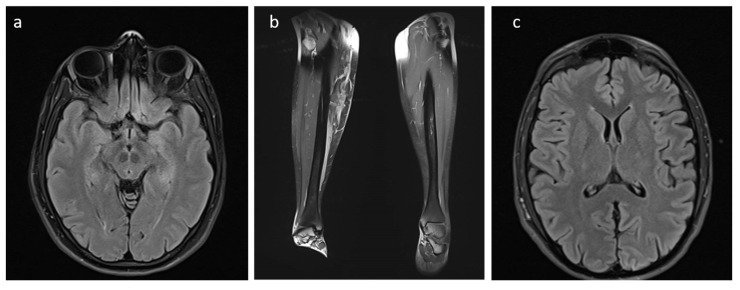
MRI of patients 1 (a,b) and 2 (c). Patient 1: Hyperintensity lesions with nonspecific significance on T2/FLAIR brain MRI in the thalamus (a). Presence of pathological tissue in the anterior compartment of the right leg. Patient 2: Hyperintensity areas on T2/FLAIR brain MRI in both lenticular capsule and thalamic regions (c).
